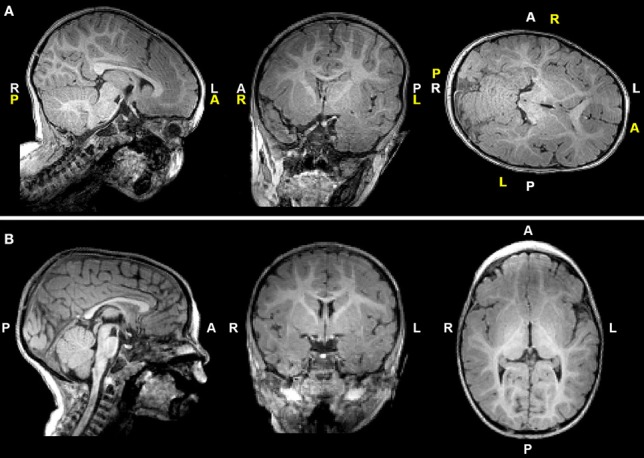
T1-weighted images of a representative full-term infant before (A) and after (B) manual realignment using Freeview (see Methods). Header information (white letters) is inaccurate in (A) due to rotated and tilted head position; corrected labels are shown in yellow letters for reference. A, anterior; L, left; P, posterior; R, right.
